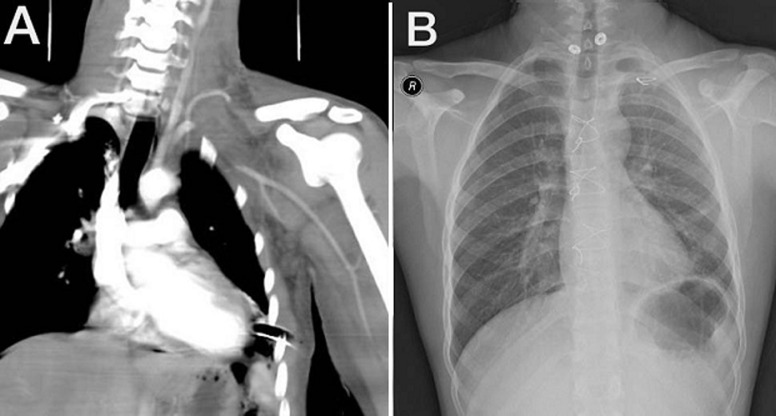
A) postoperative computed tomography angiogram of neck and upper limb; B) plain chest X-ray, posteroanterior view during the outpatient clinic visit
